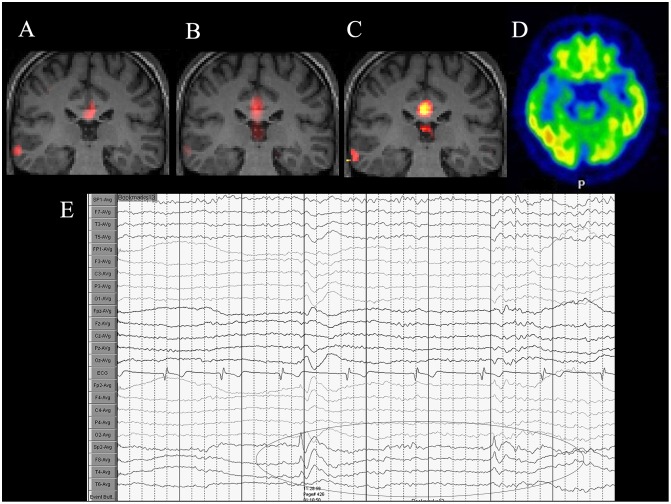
A 38-year-old man diagnosed with right lateral temporal lobe epilepsy. (A) Coronal fALFF, (B) ALFF, and (C) ReHo reveal abnormal activation in the right lateral temporal lobe. An area of activation could also be found in the corpus callosum, which is considered a part of the default mode network. (D) The corresponding ^18^F-FDG PET/CT image reveals low FDG uptake in the right lateral temporal lobe region. (E) VEEG depiction of interictal epileptiform discharges of right temporal lobe.
